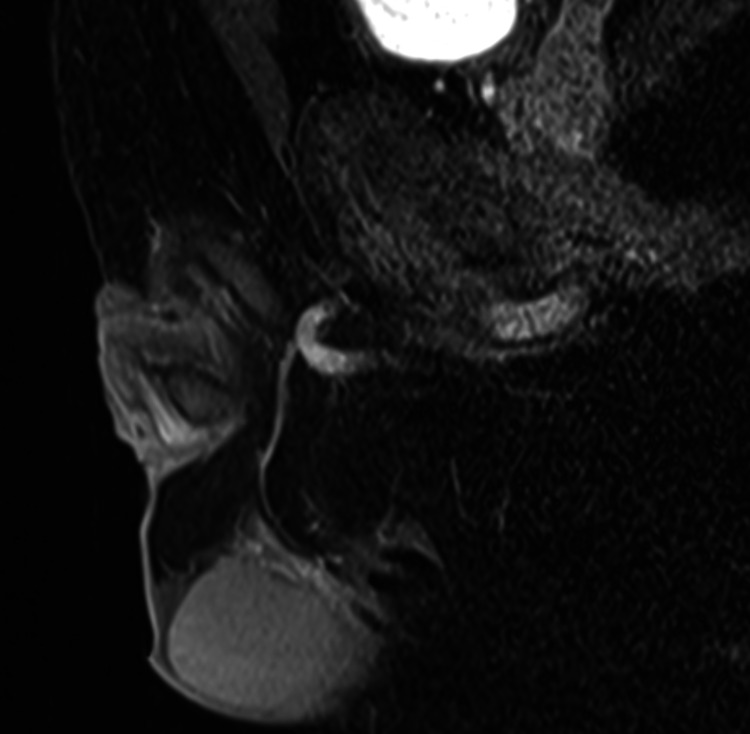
T2-weighted MRI shows no abnormal findings in the left testicle after treatment.
